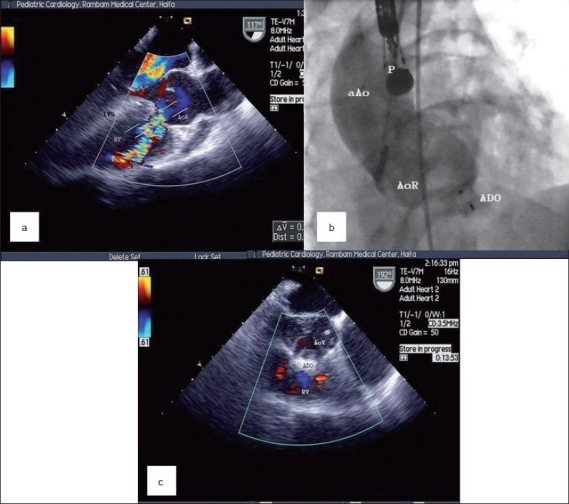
(a) TEE with color Doppler showing fistula opeining into the right ventricle (RV); (b) Full deployment of the 12 × 10 mm Amplatzer Duct Occluder (ADO) in the fistula after release; (c) TEE of the ADO *in situ* occluding the shunt (IVS, intraventricular septum; AoR, aortic root; F, fistula; aAo, ascending aorta; P, probe of TEE)
